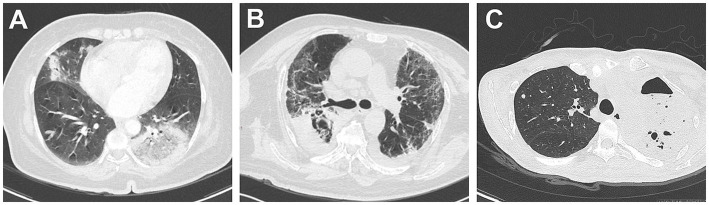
Characteristic chest CT imaging findings of pulmonary mucormycosis. (A) The reverse halo sign is observed in the left lower lobe, alongside multiple nodules exhibiting halo signs in the right middle lobe. (B) A cavity with septations in the right lower lobe, which evolved from a reverse halo sign, is accompanied by bilateral pleural effusions. (C) Small nodules are noted in the right upper lobe, with extensive consolidation and cavity formation in the left lung.
